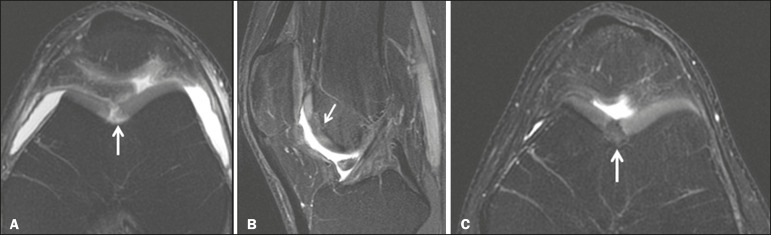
A 26-year-old man submitted to microfracture. T2-weighted fat-saturated MRI sequences. Preoperative axial image (A) showing a deep chondral lesion in the center of the femoral trochlea (arrow). Follow-up imaging at seven months of the procedure: sagittal and axial images (B and C, respectively), showing the microfracture trajectories, together with subchondral edema (arrows) and fibrocartilaginous tissue filling the chondral lesion site.
